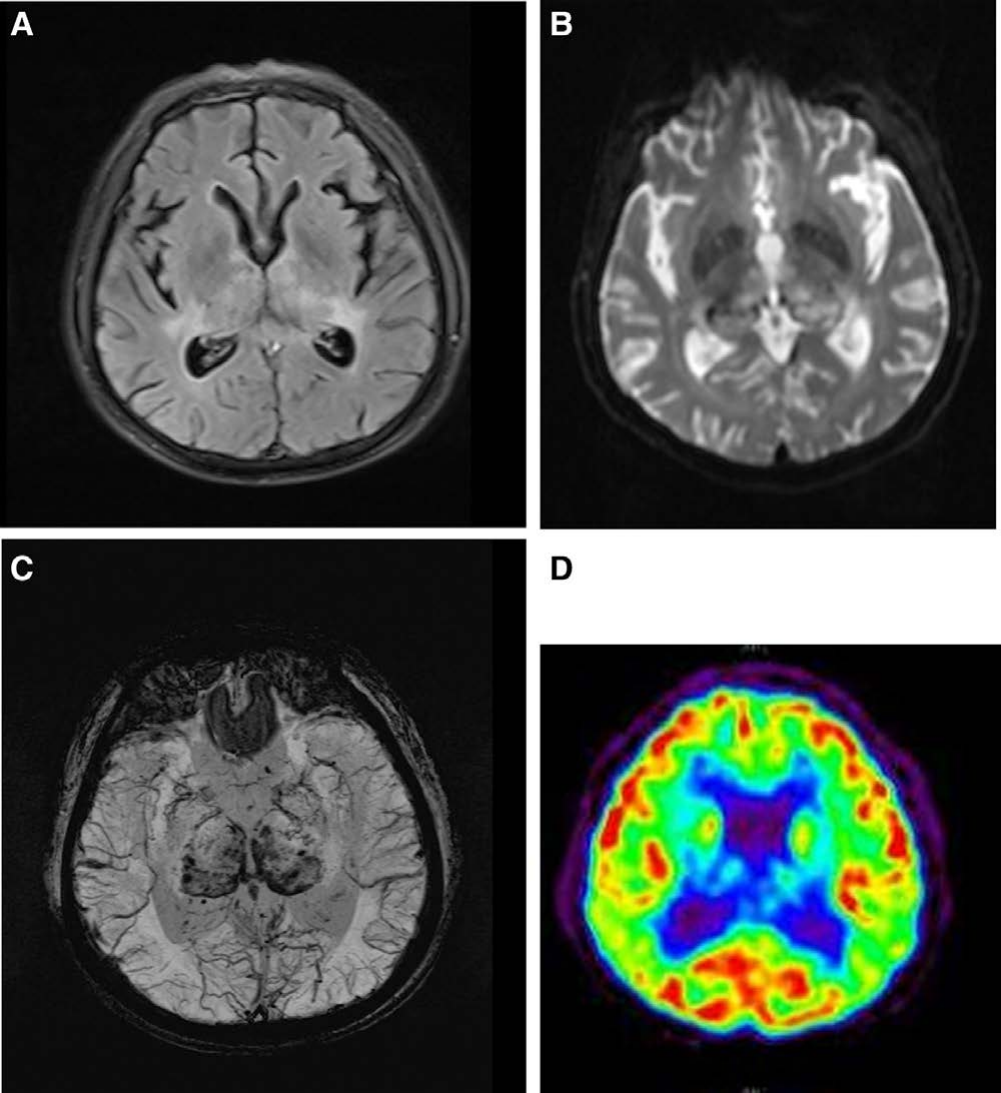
(A) Plain magnetic resonance imaging (MRI) scan result showed that an abnormal signal was symmetrical in the bilateral thalamus. (B) Diffusion weighted imaging (DWI) result showed that an abnormal signal was symmetrical in the bilateral thalamus. (C) Craniocerebral susceptibility weighted imaging (SWI) indicated bilateral thalamus microhemorrhage and alterations in venous dilation. (D) Positron emission tomography-computed tomography (PET-CT) examination revealed decreased symmetrical density of the bilateral thalamus and lowered glucose metabolism.
